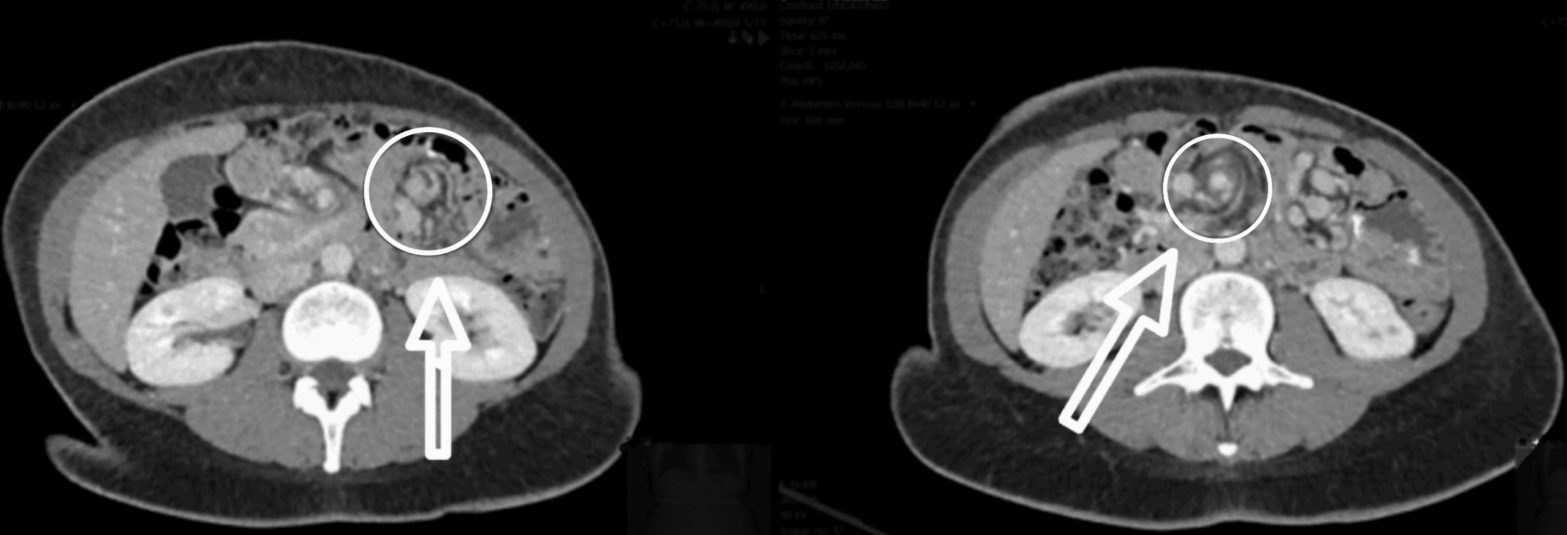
Case 4 - Axial CT scans showing progressive mesenteric swirling suggestive of Petersen’s hernia Two consecutive axial slices of a contrast-enhanced abdominal CT. The white circle delineates the twisted mesenteric vessels and fat (swirl sign), consistent with internal hernia. The white arrows highlight the progressive rotation of the mesentery from one slice to the next (Image A to Image B). These radiologic features support the diagnosis of Petersen’s hernia, even in the absence of overt bowel obstruction.
